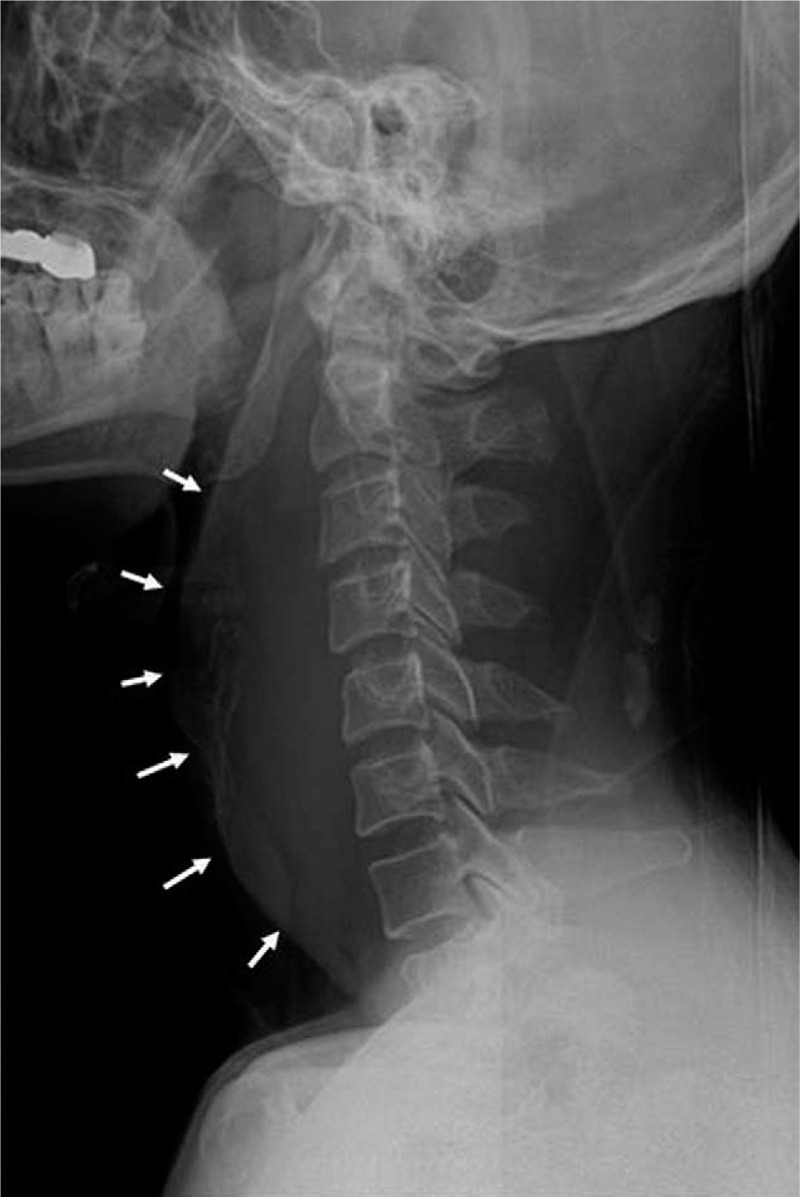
Initial lateral neck X-ray shows marked widening of prevertebral space (arrows).
